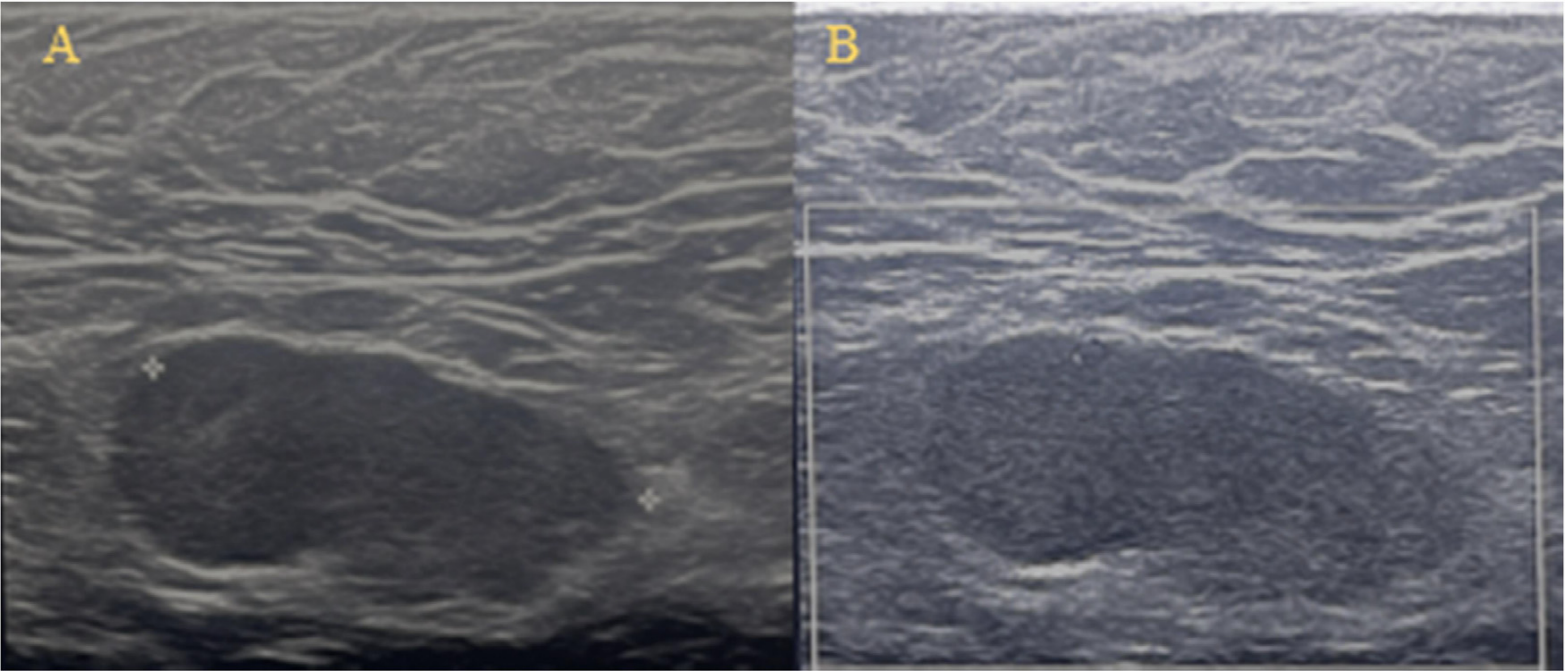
Ultrasound. Irregularly hypoechoic oval-shaped formation in the right axilla (measured about 2.89 cm) (A); No significant increase in vascularization was observed on colour Doppler ultrasound (B).
